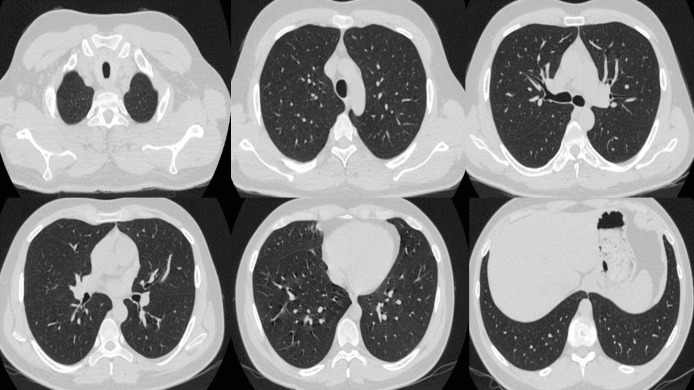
Figure 3. In the axial CT images obtained during
